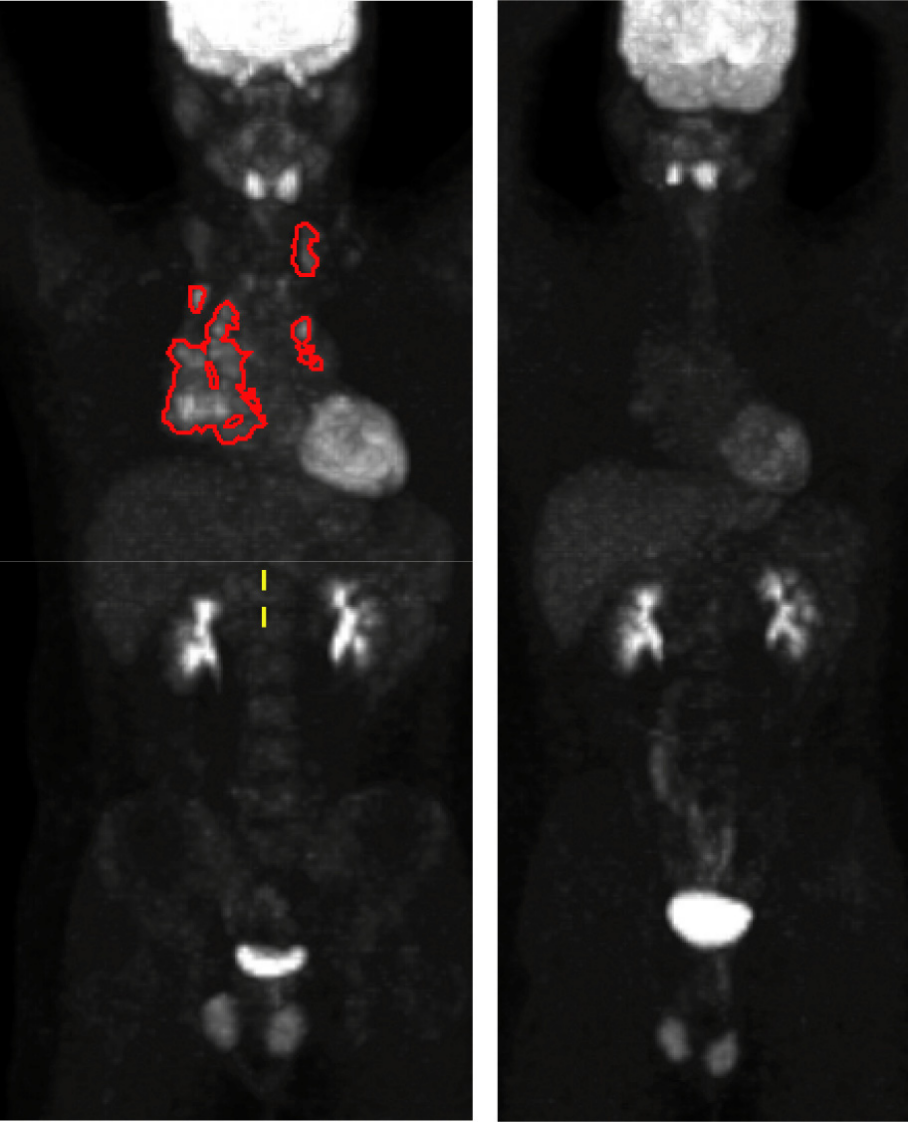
Maximum intensity projection view of ^18^F-fluorodeoxyglucose-positron emission tomography image with overlay of segmented metabolic tumor volumes (MTV). This is a representative pre-treatment PET image (left; MTV = 94 mL, SUVmax = 8.9, SUVmean = 3.4, and iSUV = 319.8) and interim-treatment PET image (right; absolute value and calculated MTV = 0 mL, SUVmax = 0, SUVmean = 0, and iSUV = 0).
